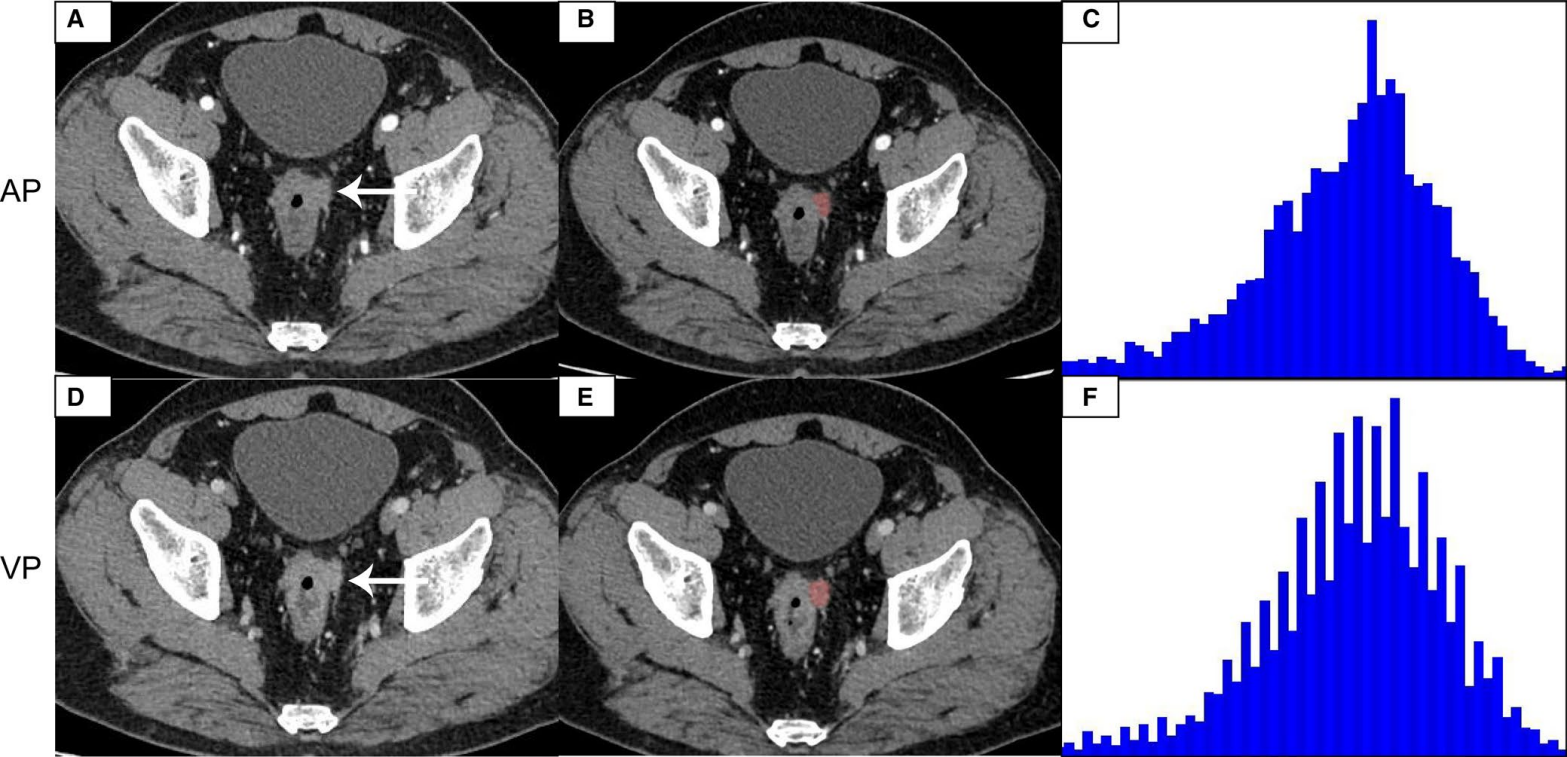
A 56‐year‐old male with G3 R‐NETs. The tumor was significantly enhanced on the arterial phase image (A), and the degree of enhancement is reduced on the venous phase image (D). The tumor region of interest (ROI) was localized on axial CT images at arterial phase (B) and venous phase (E). CT histogram at arterial phase (C) and venous phase (F) were shown
